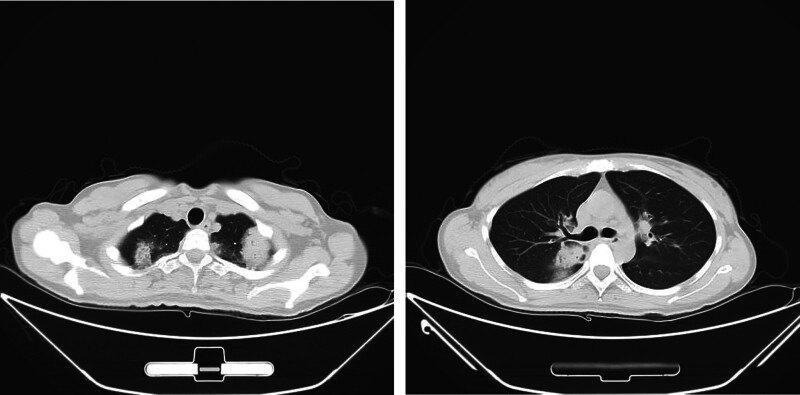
A repeat CT scan showed further expansion of the patchy lung shadow.
